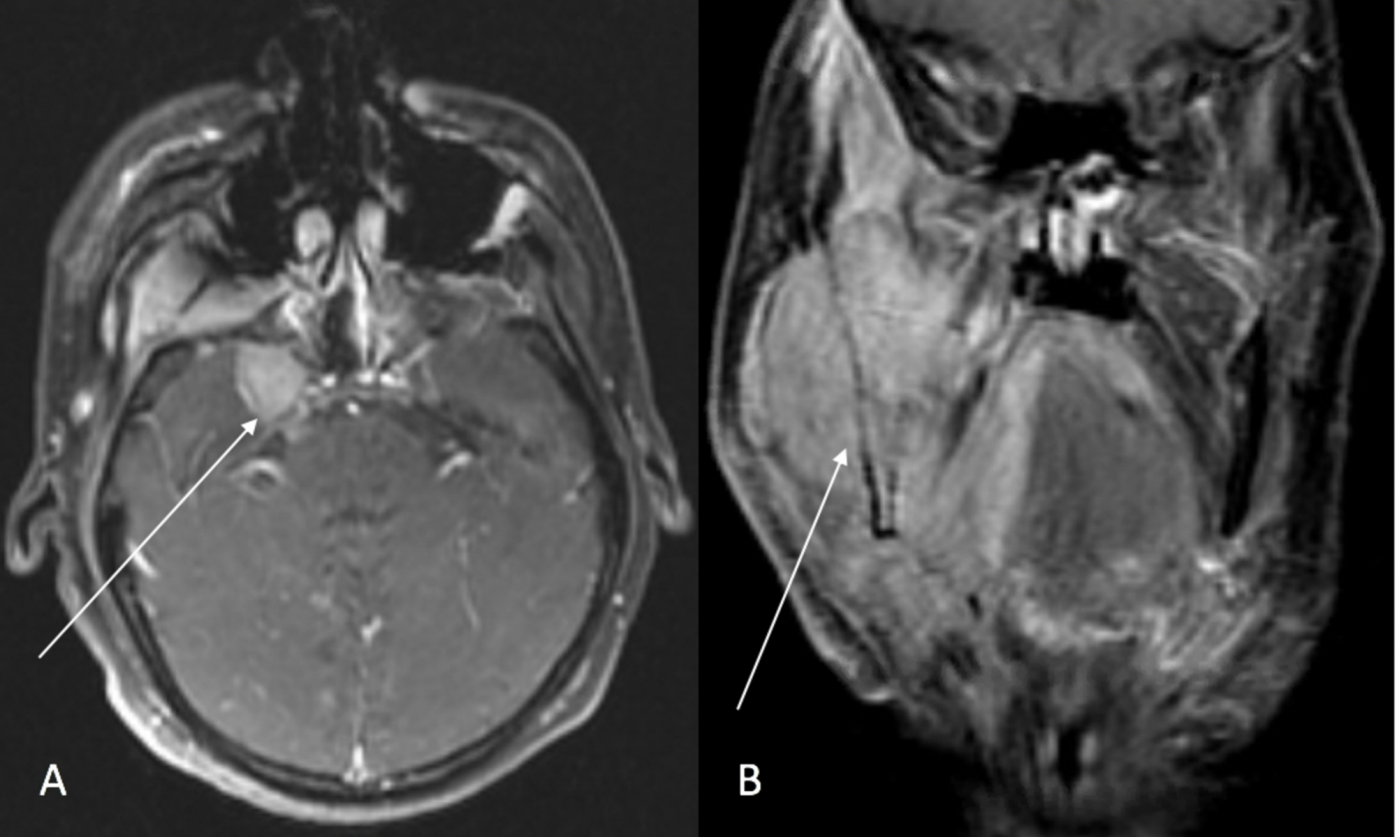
MRI brain and neck with contrast at presentation The white arrow in pane A (axial view) points to the involvement of right Meckel's cave and cisternal segment of the right trigeminal nerve by the tumor. The white arrow in pane B (coronal view) shows the tumor invading into the right masticator space, infrazygomatic space and the oral cavity.
